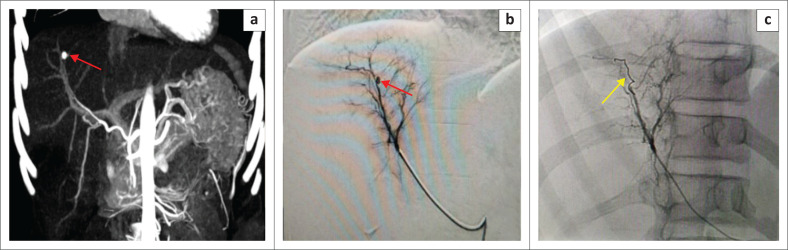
Coronal CT maximum intensity projection image (a) shows a well-defined pseudoaneurysm in close proximity to the segment VIII artery (red arrow a). Angiography via a microcatheter (b) shows a small pseudoaneurysm arising from the segment VIII artery (red arrow b) which was embolised (c) by placing a microcoil across the neck of pseudoaneurysm using the sandwich technique (yellow arrow c).
